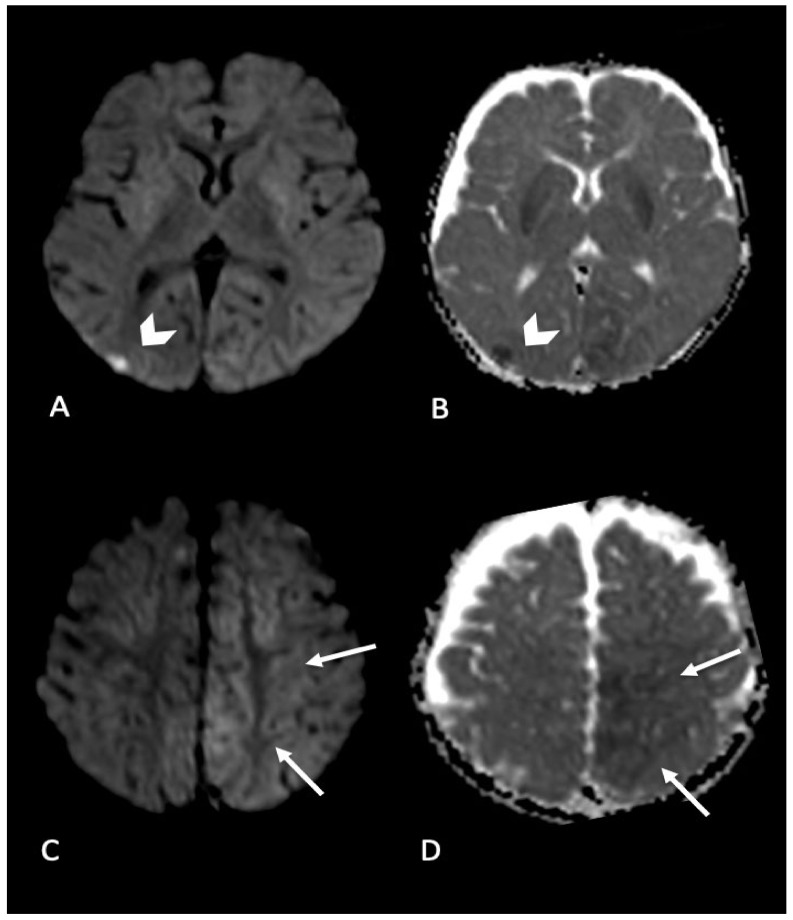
Infant found with right side focal seizures with secondary generalization. Bruises on the right ear, retinal hemorrhages and subdural hemorrhages. MRI performed in the emergency department showed bilateral parenchymal injury. In particular, in (A,B) (axial DWI and ADC map) diffusion weighted imaging revealed focal area of restricted diffusion in right occipital cortex (arrow’s head); (C,D) (axial DWI and ADC map) showed areas of restricted diffusion in left mesial parietal cortex (arrows).
